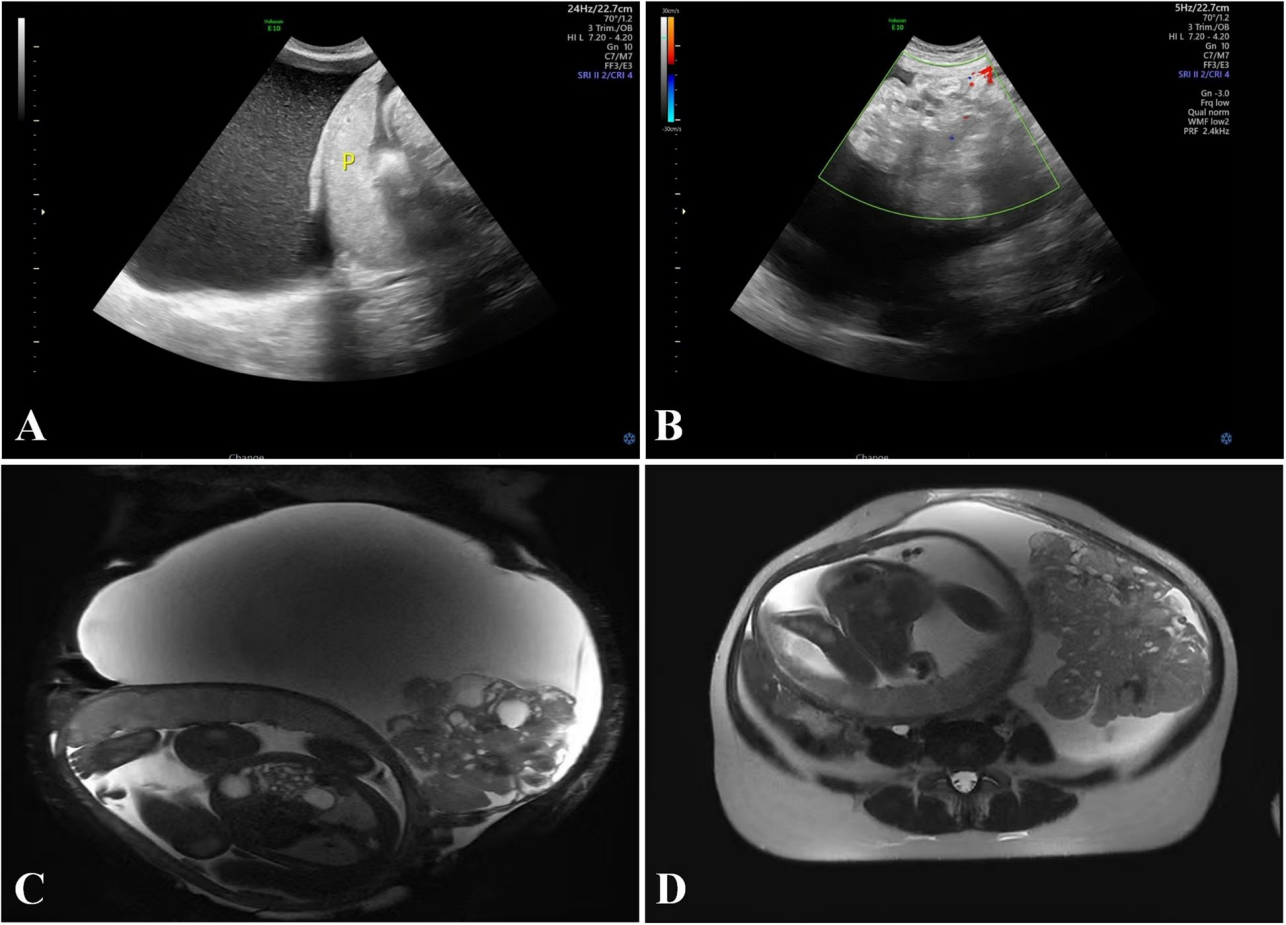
The ultrasound and MRI images of the tumor. A and B, sonographic examination demonstrated there was a 25.0 × 15.0 × 13.7 cm cyst in the left adnexal area, and the cyst was found to have a 15.7 × 11.3 × 14.1 cm heterogeneous echogenicity, which was irregular cauliflower-shaped and had strong blood flow. p indicate placenta. C and D, Abdominal magnetic resonance imaging (MRI) revealed a 30 × 17.4 × 18.9 cm cystic and solid mass on the left side of the pelvic cavity, and the solid component was irregular-shaped and cauliflower-shaped appearance
